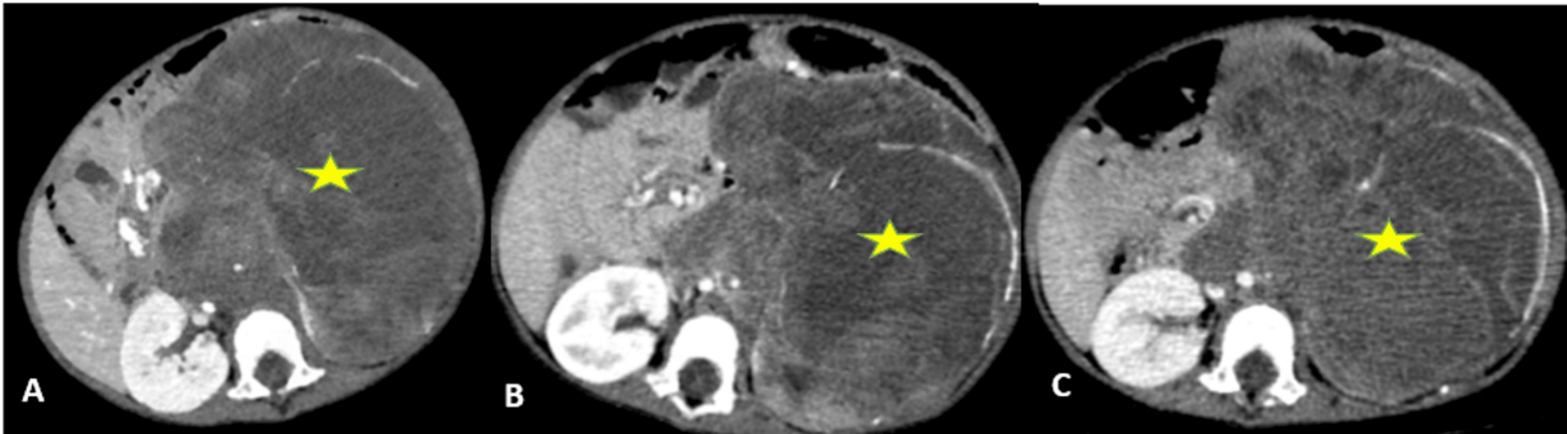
Axial slices of the initial abdominopelvic CT scan (A), first follow-up (B), and second follow-up (C) images of the retroperitoneal mass (asterisk), showing a slight size reduction.
